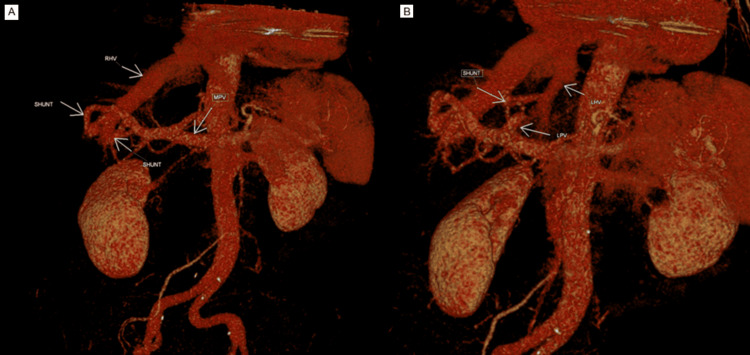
(A, B) CT shows the presence of shunts in both lobes of the liver RHV: right hepatic vein; MPV: main portal vein; LHV: left hepatic vein; LPV: left portal vein; CT: computed tomography
